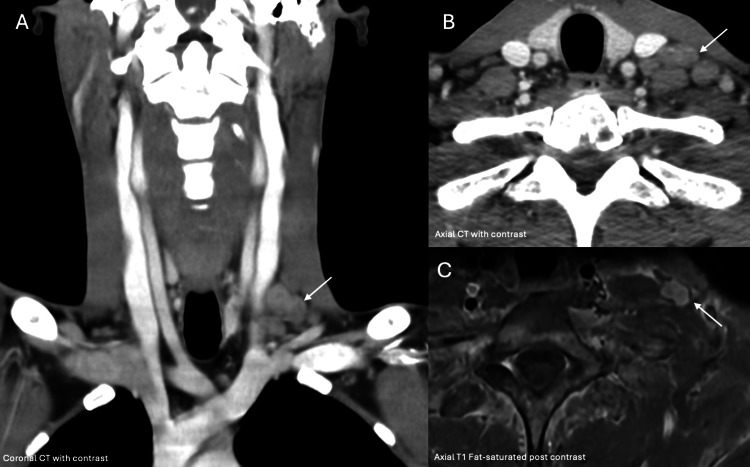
Contrast-enhanced neck CT (A, B) and cervical MRI (C) demonstrating a left supraclavicular nodal conglomerate, including involvement of Virchow’s node (arrows).
